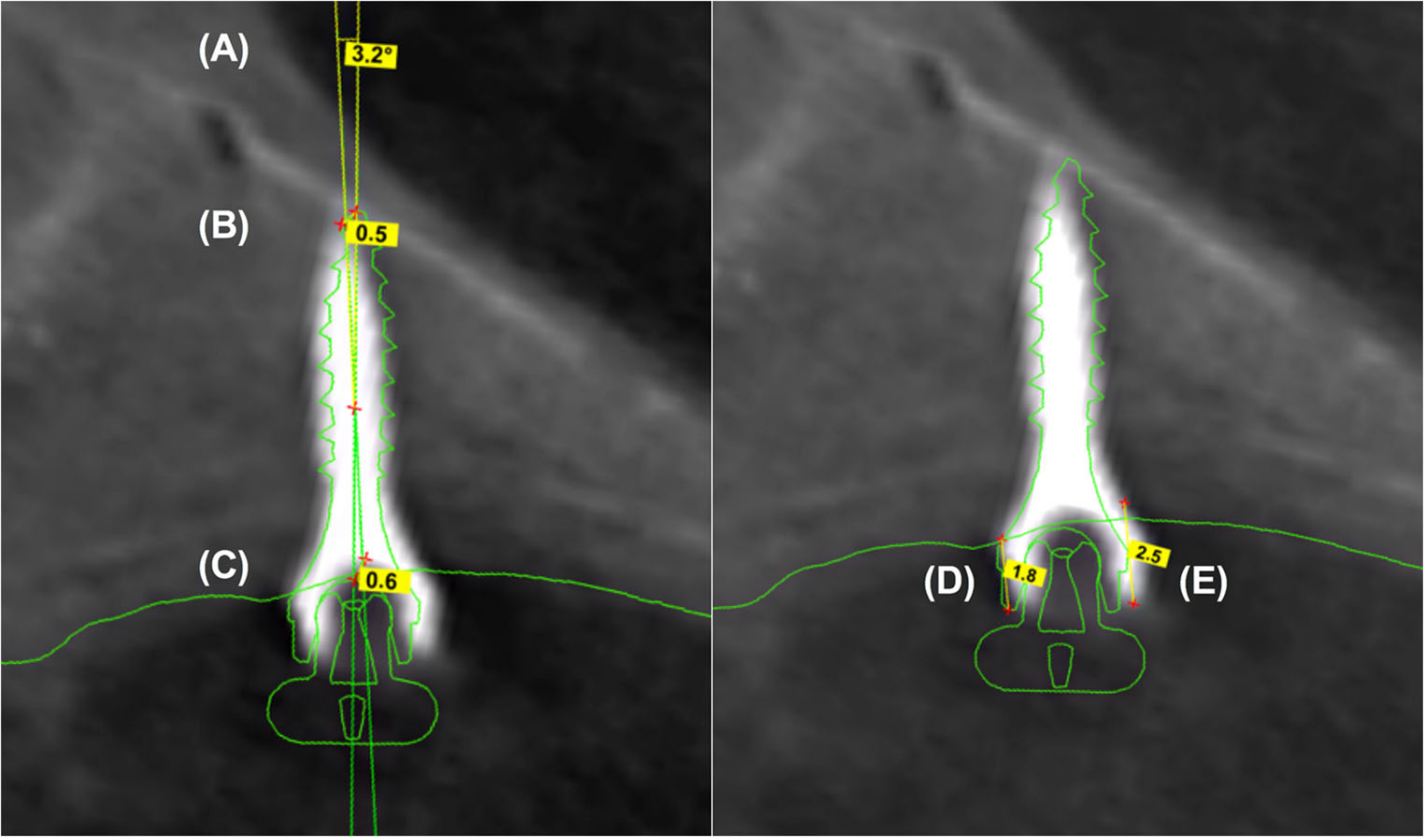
Three-dimensional measurements between the superpositioned files of the deviations of the implant axis (a), implant tip (b) and implant shoulder (c). Evaluation of the distance between the vertical implant position of the anterior (d) and posterior implant shoulder (e) to the contact of the gingiva at the implant. Measurements of the anterior and posterior vertical implant position were performed twice: during preoperative planning (d) and during postoperative CBCT scans (e)
